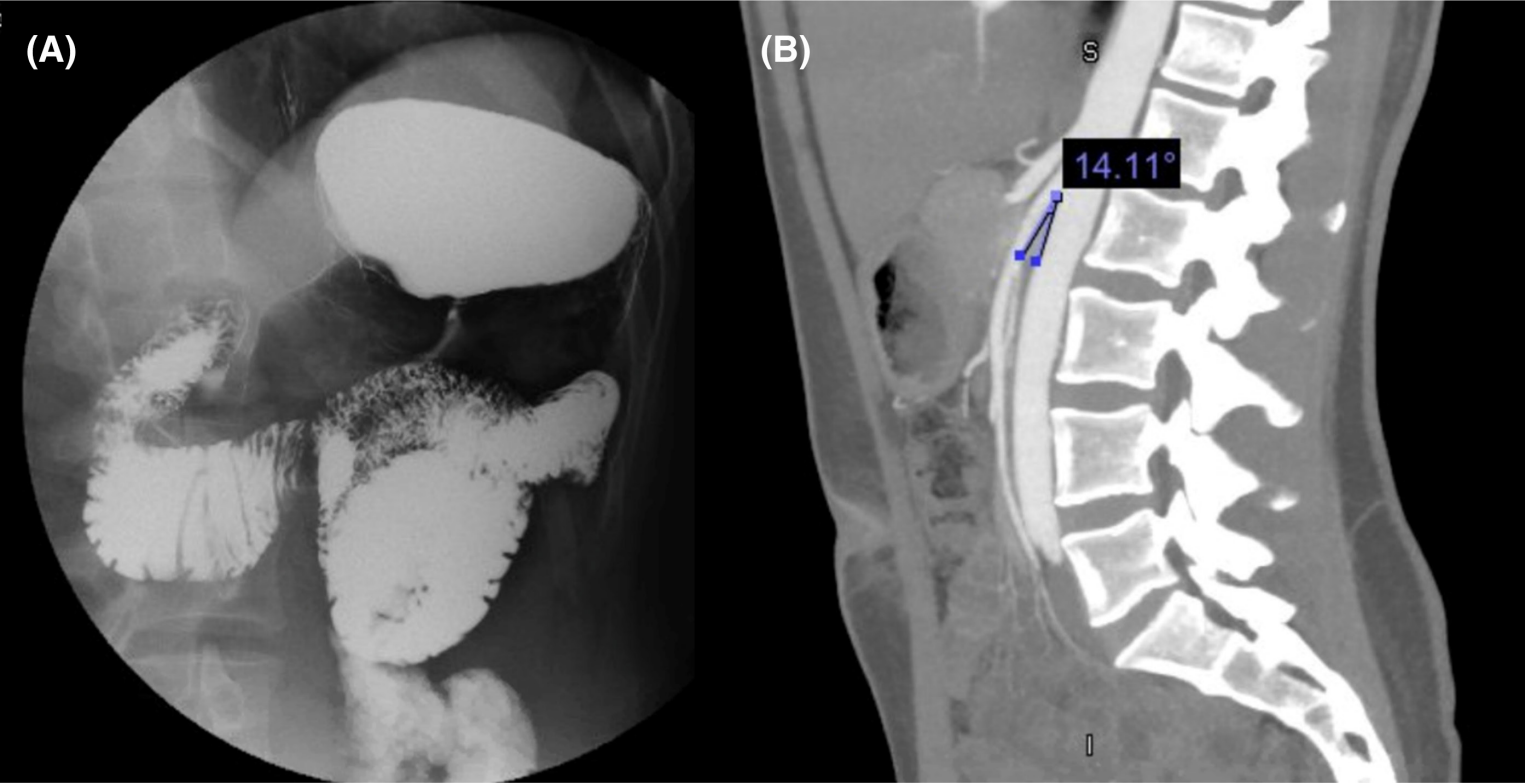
(A) Preoperative upper GI imaging demonstrating narrowing of the third portion of the duodenum with diminished transit of contrast (B) Preoperative CTA demonstrating a narrowed aortomesenteric angle of 14.11 degrees, consistent with the diagnosis of SMAS.
